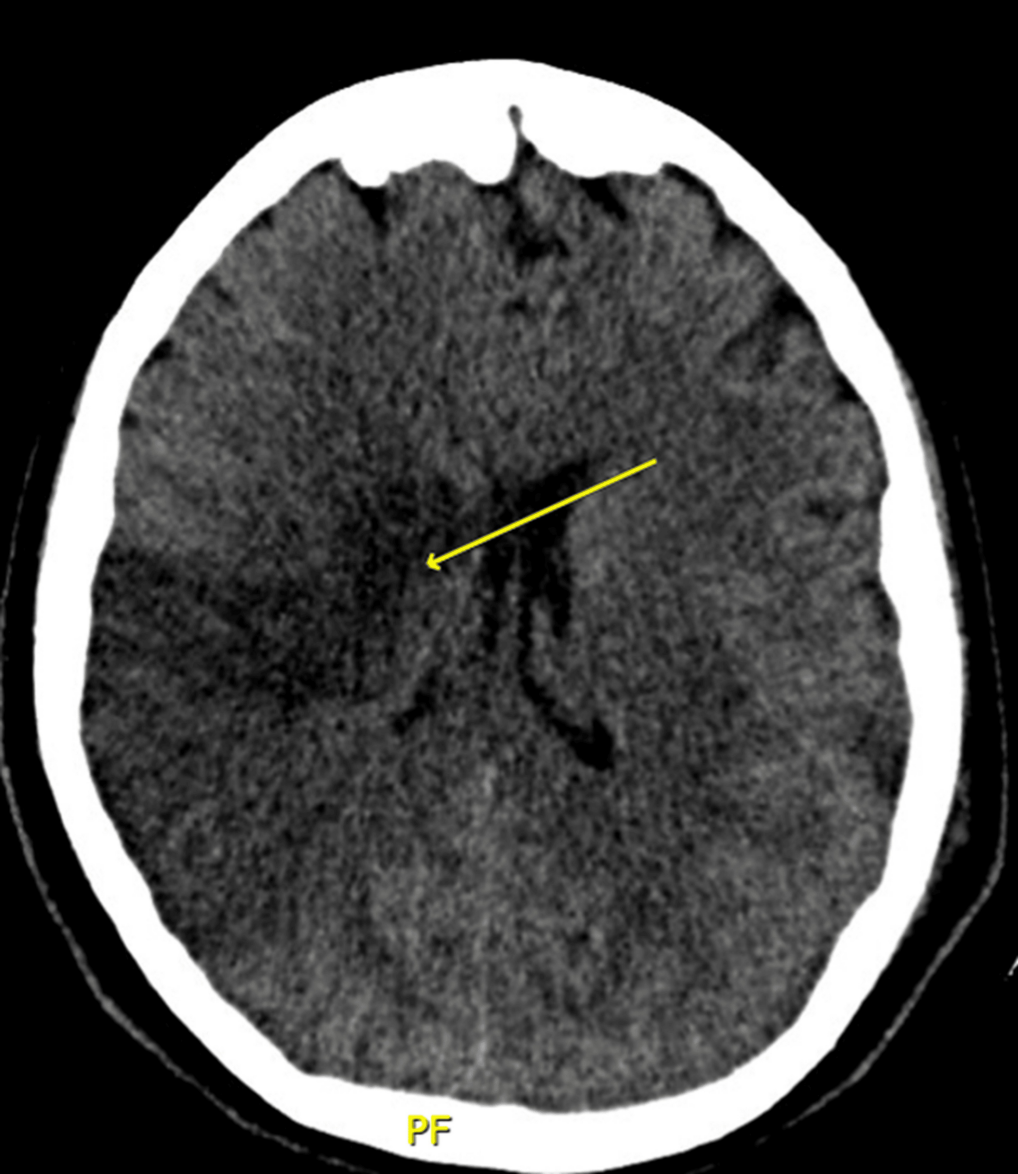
Control brain computed tomography (CT) Brain CT demonstrating a large hypodense area involving the right frontoparietotemporal region (arrow), with associated cytotoxic edema.
